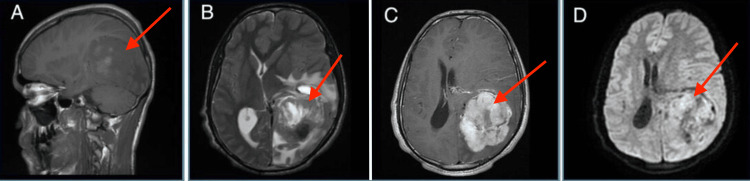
Brain MRI showing a 5 x 5 x 4 cm multilobulated mass in the occipital horn of the left lateral ventricle with intense contrast enhancement, perilesional edema, and mass effect, but no dural tail sign, with a central necrotic area (red arrows). (A) Sagittal T1 sequence showing hypointense to isointense T1 signal. (B) T2 sequence showing a heterogeneous lesion with both hypo- and hyperintense T2 signals. (C) Axial T1 sequence with gadolinium contrast showing intense enhancement with mass effect and midline shift. (D) DWI sequence showing a heterogeneous lesion with a low ADC index. DWI: Diffusion-weighted imaging, ADC: Apparent diffusion coefficient.
